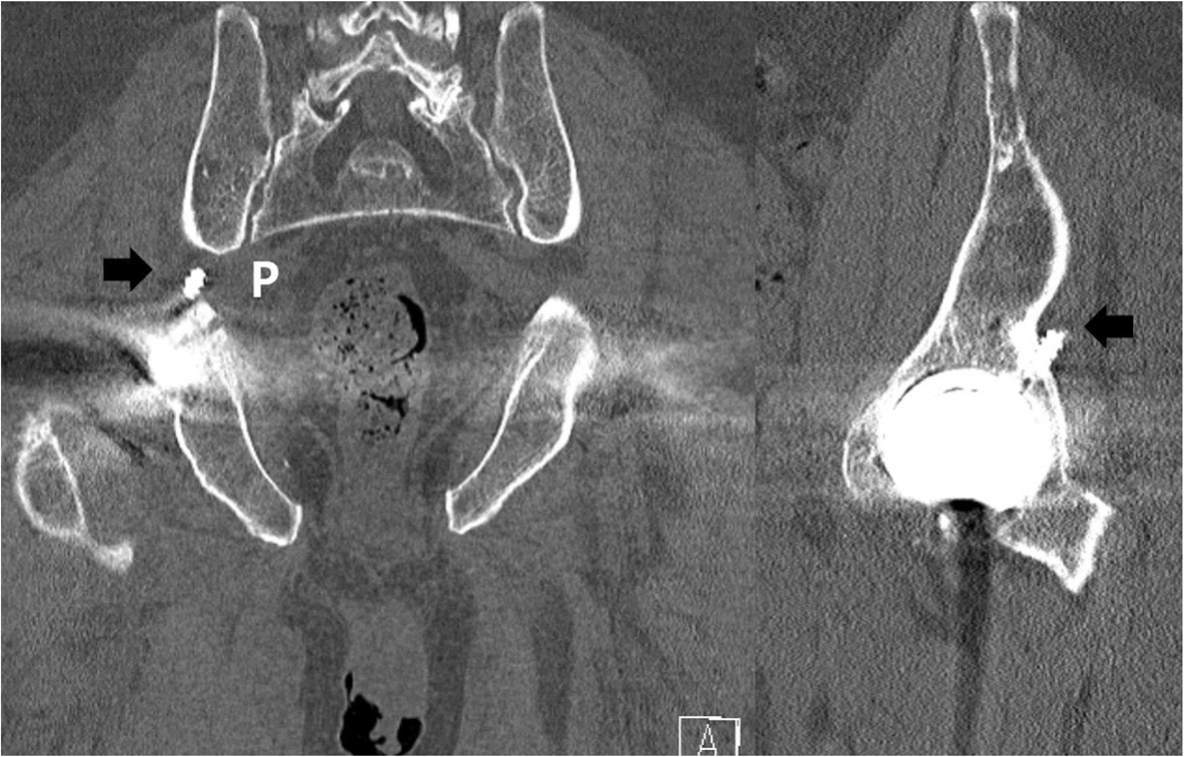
Coronal and computed tomography views of right hip showing sciatic notch area occupied by an acetabular screw (arrow). The protruded screw was placed superiorly than piriformis muscle (P)
